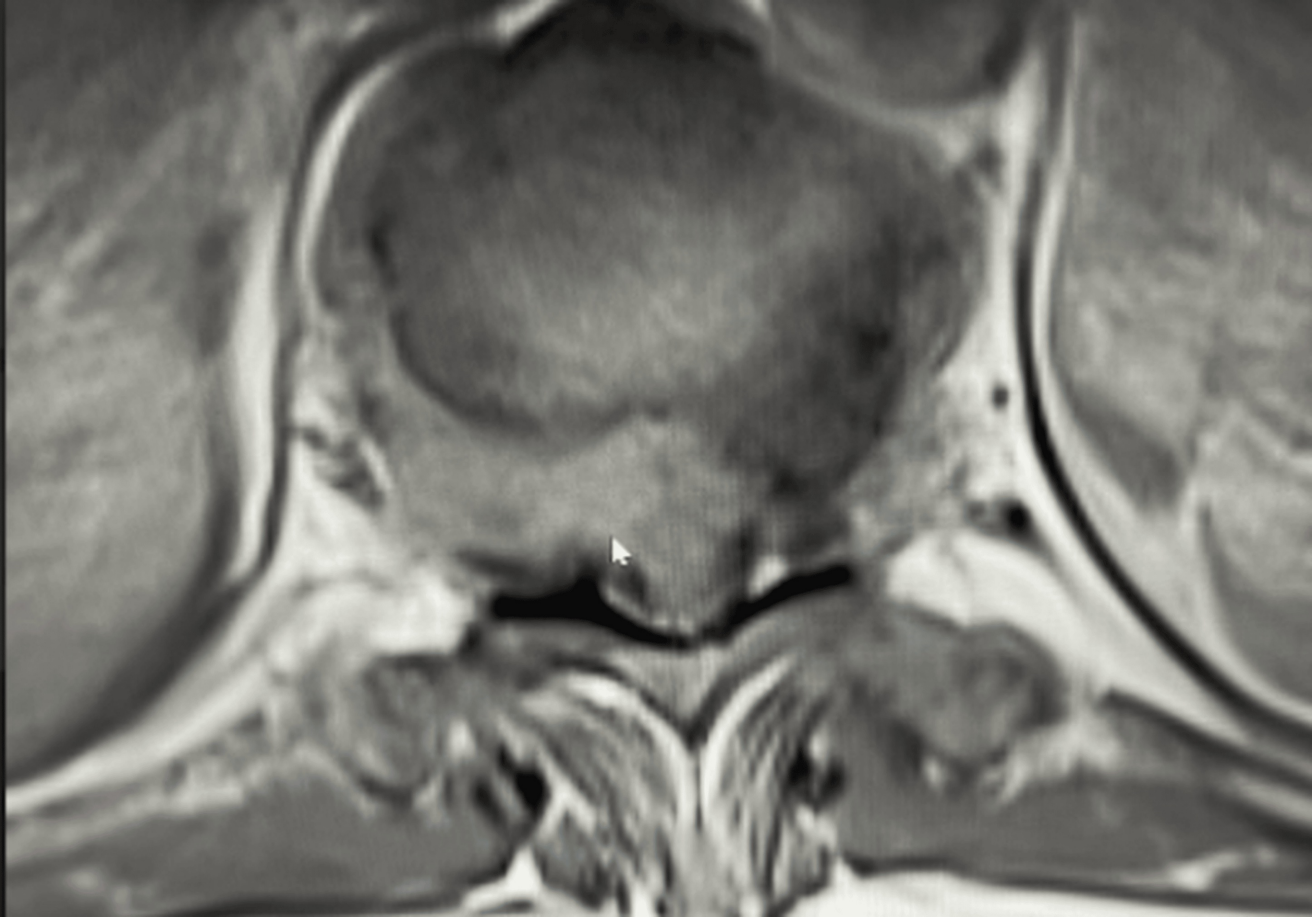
Patient 3: MRI demonstrating a mass within the thoracic cavity encroaching upon the bone-localized marrow, consistent with metastatic infiltration of the thoracic vertebral structures.
